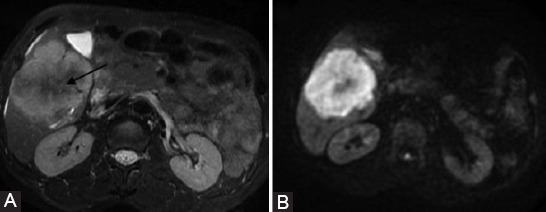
Targetoid appearance in T2WI-FS (A) and diffusion-weighted imaging [DWI] (B). A surgically confirmed combined hepatocellular carcinoma-cholangiocarcinoma (cHCC-CCA) in a 50-year-old male with hepatitis B virus infection. Concentric pattern in T2WI-FS and DWI characterized by mild-moderate hyperintensity in observation periphery with milder hypointensity in the center (arrow).
